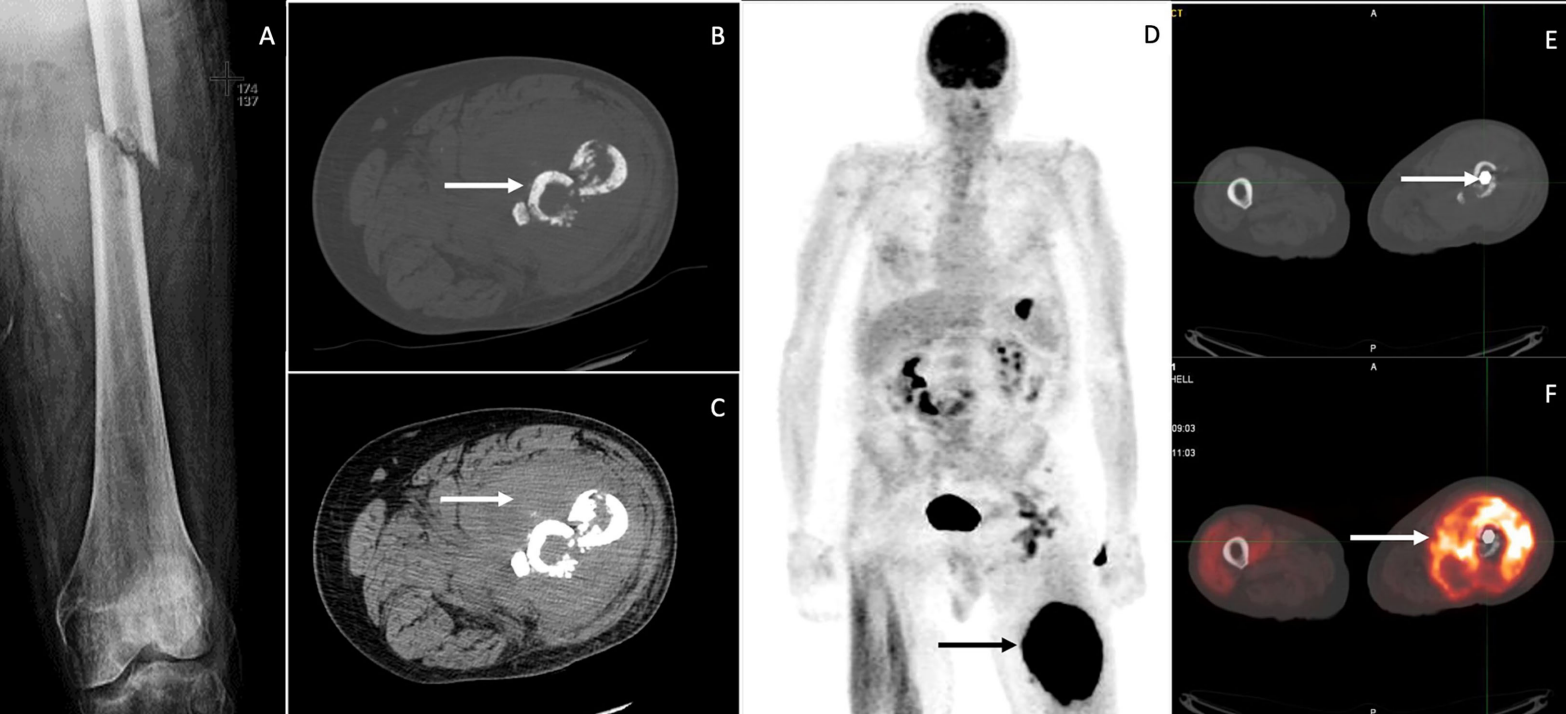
X-ray demonstrates displaced mid femoral pathologic fracture through a permeative lesion with improved alignment and diffuse osteopenia (A). Axial images from CT of the left lower extremity showing a pathologic fracture involving the left proximal femur with sclerotic and fragmented bones (arrow in (B), and a large heterogeneous soft tissue density mass surrounding the bone fragments (arrow in (C). Whole-body image from the FDG PET/CT scan (D) shows intense hypermetabolism in the left thigh mass, consistent with neoplastic involvement. Other foci of increased uptake were thought to be non-neoplastic. The pathologic fracture has been internally fixed with an intramedullary nail (arrow in (E), and the axial fused PET/CT image shows intense hypermetabolism in the mass surrounding the proximal femur (arrow in (F).
